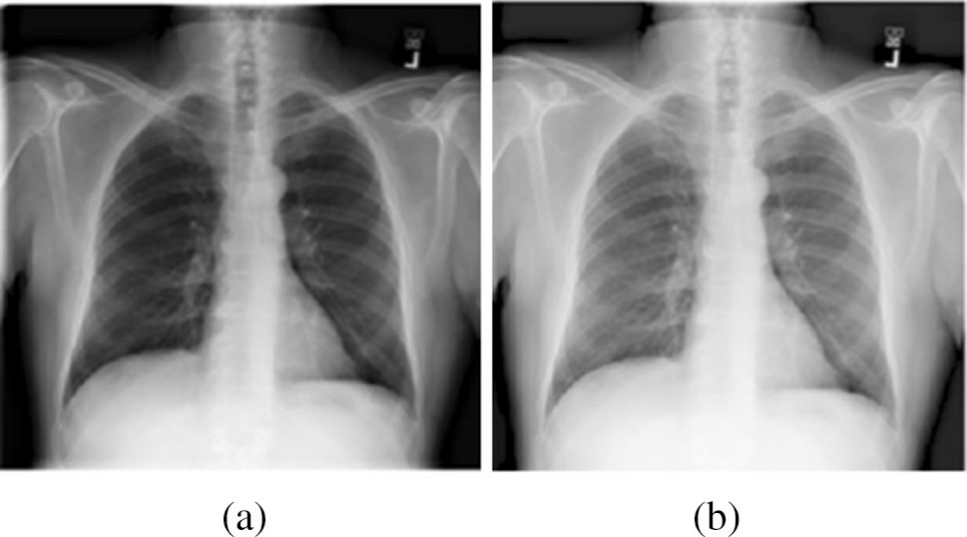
Samples X-ray image for before (a) and after (b) applying gamma correction
